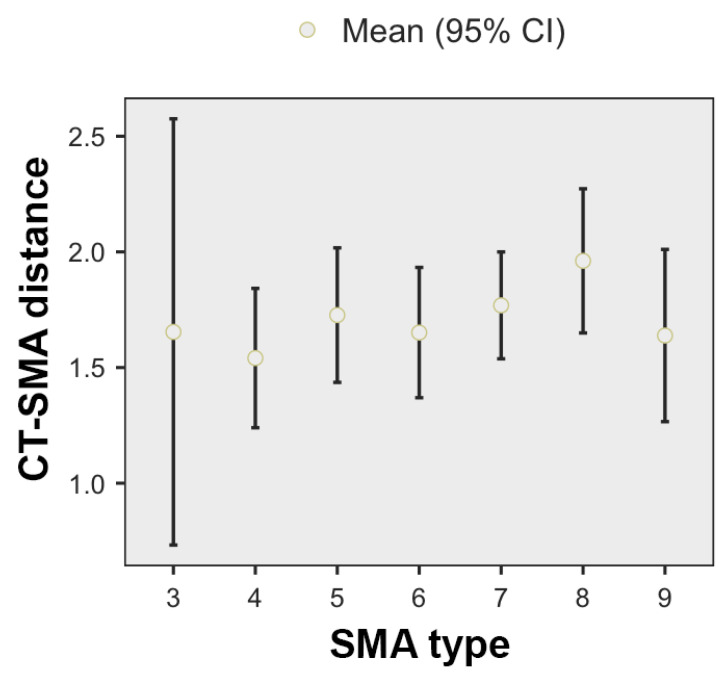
CT–SMA distance, depending on the SMA type.
